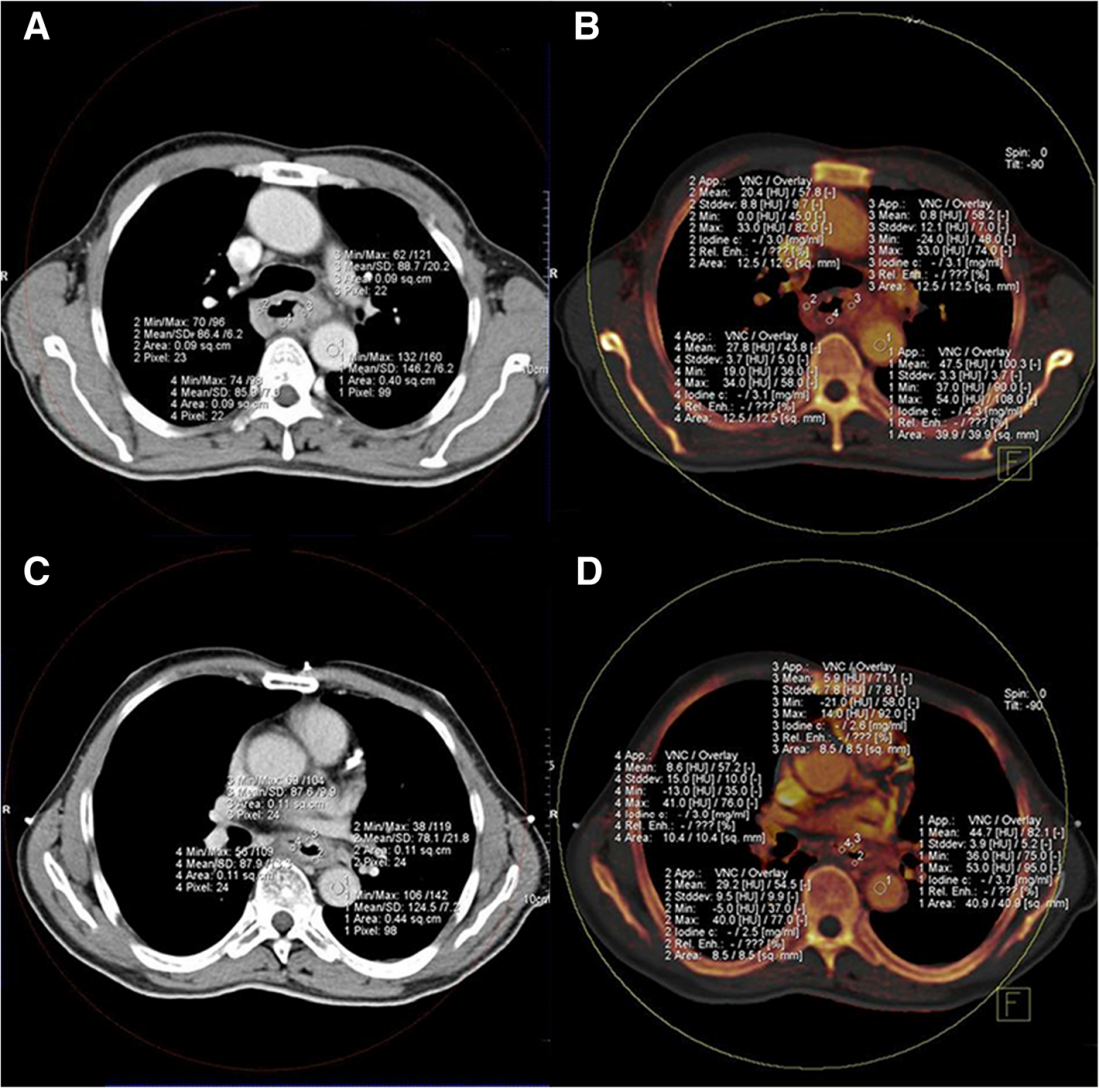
CT images of esophageal cancer before and after treatment. a The mucosal and aortic CT value of esophageal cancer before treatment; b The mucosal and aortic iodine values of esophageal cancer before treatment; c The mucosal and aortic CT value of esophageal cancer after treatment; d The mucosal and aortic iodine values of esophageal cancer after treatment
